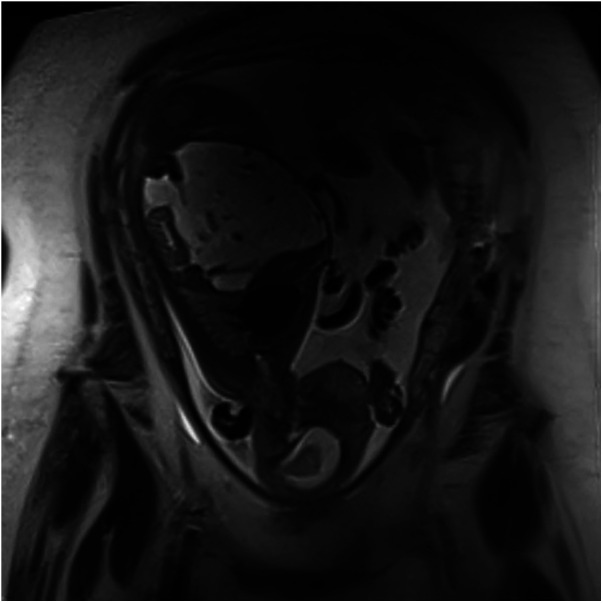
Coronal MRI scan, T2-weighted sequence, showing a hyperintense multiloculated cystic lesion (Bambino Gesù Children's Hospital, Rome, Italy).
